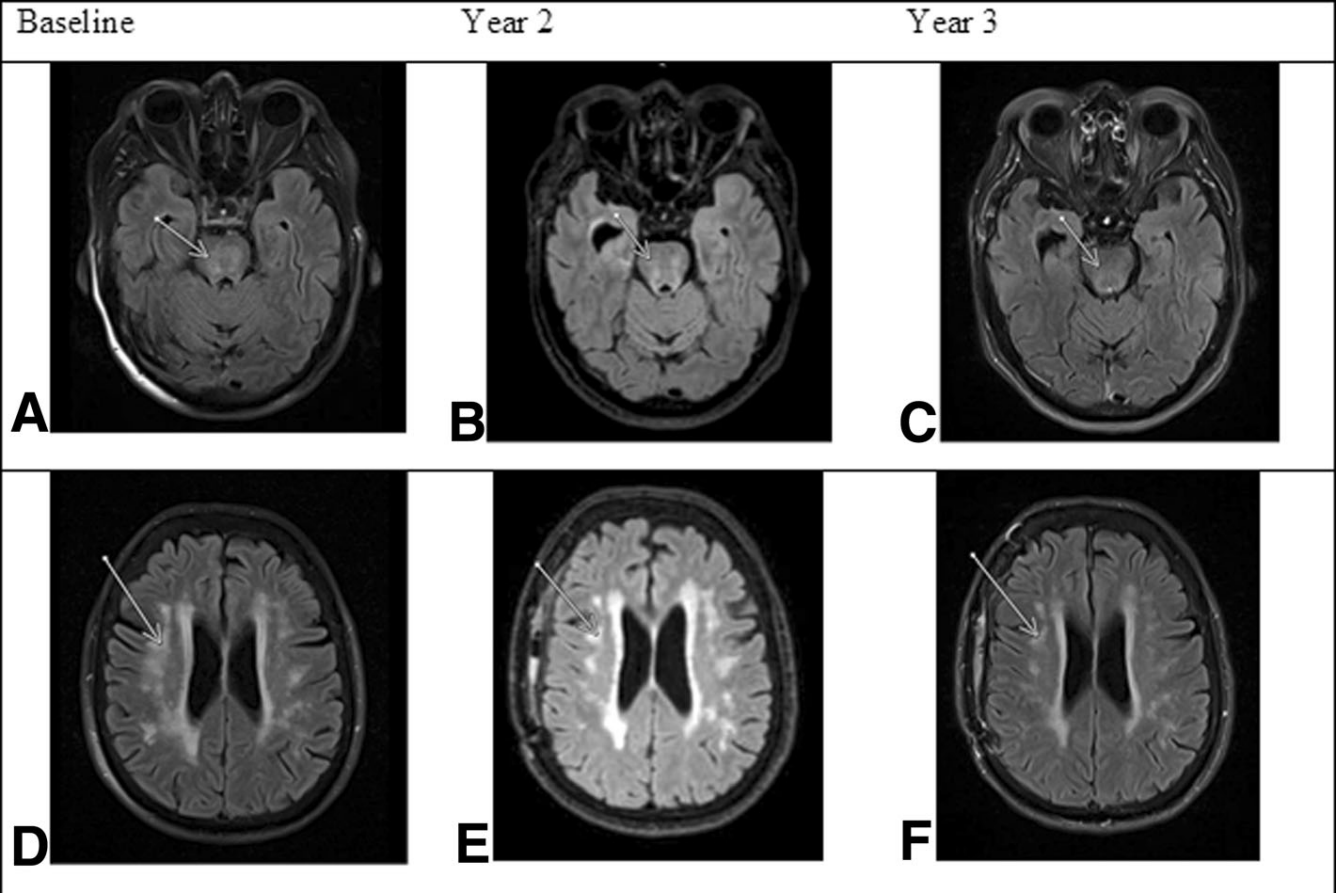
Resolution of small vessel ischemia following the pituitary adenoma resection. Legend: Imaging studies obtained before and after treatment. Axial and sagittal FLAIR MRI images show the progressive resolution of microvascular ischaemic changes in the brainstem and cerebral hemispheres over time. Baseline (Panels a and d), at 2 years (Panels b and e) and at 3 years after resection of pituitary adenoma (Panels c and f)
